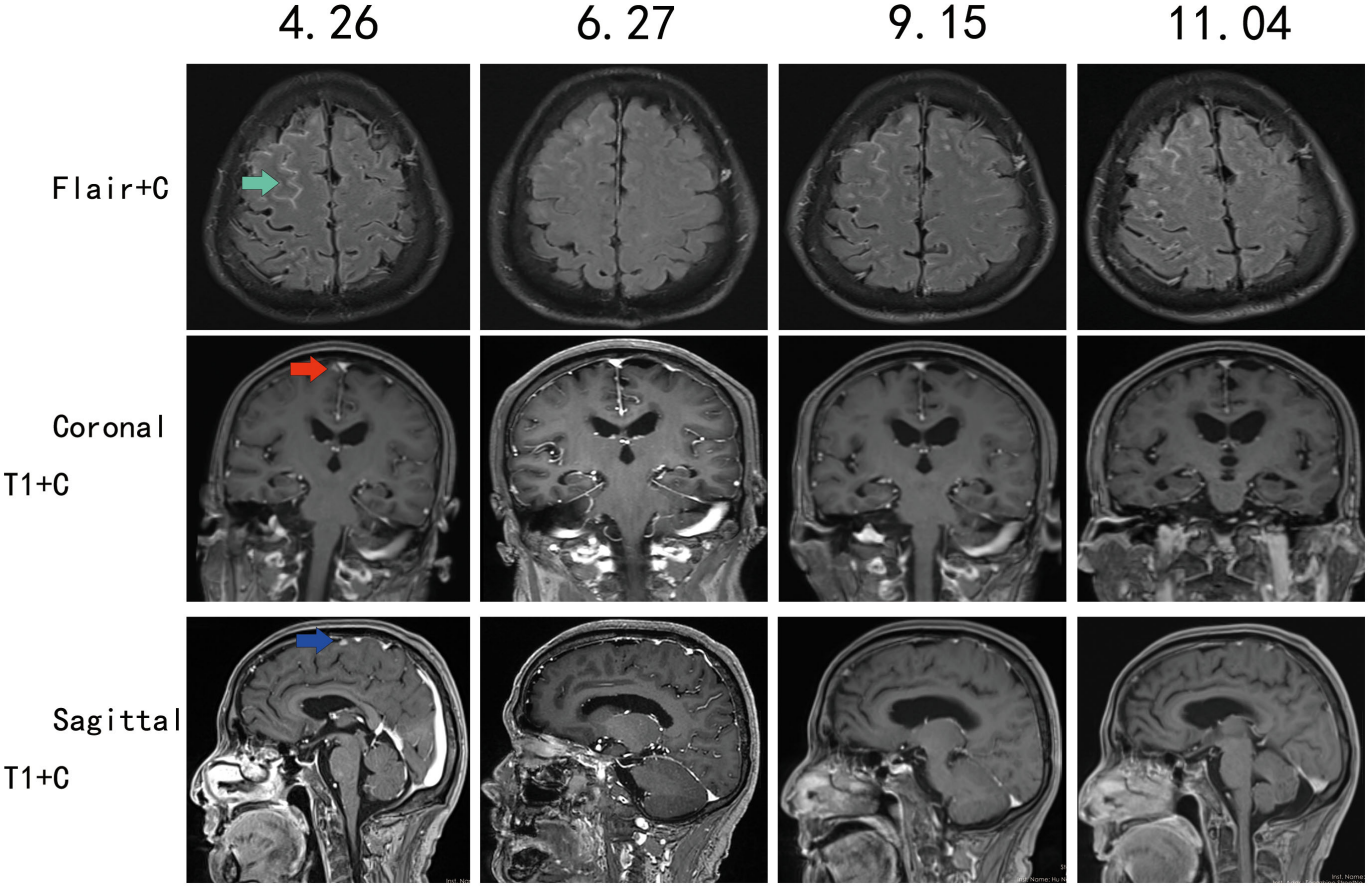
Contrast-enhanced MRI follow-up of the leptomeninges, with follow-up dates on (April 26, June 27, September 15, November 4).
